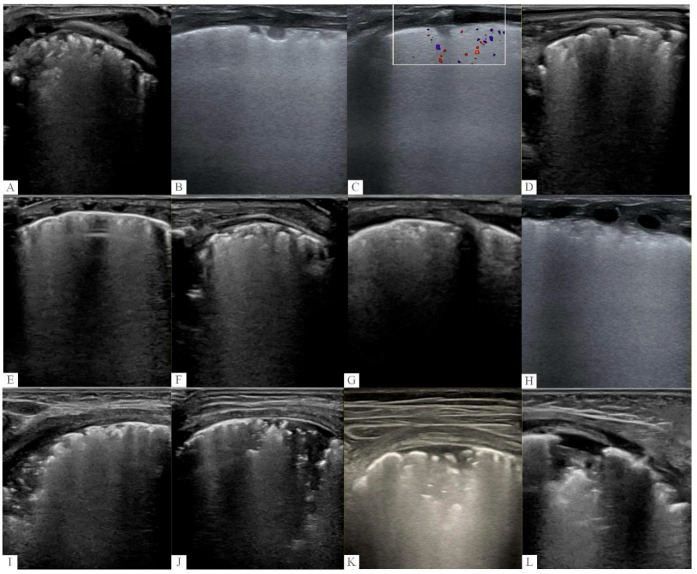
Other LUS appearances of infants with moderate–severe BPD. (A–D). In infants with moderate–severe BPD, irregular patch-like anechoic or weakly echoic appearances on the pleura surface were observed, and no blood flow was detected in the anechoic areas on color Doppler. (E–H). Scattered speckled or patch-like hyperechogenicity below the pleura was observed. (I,J). Lung consolidation accompanied by air bronchograms (>0.5 cm) was observed. (K,L). Consolidation accompanied by pleural effusion was also found in a few patients, although the difference was not statistically significant.
